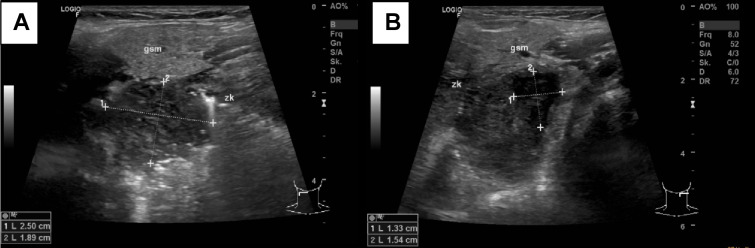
Transverse sonographic images showing (A) an enlarged heterogeneous right-sided tonsil without signs of an abscess and (B) an enlarged heterogeneous left-sided tonsil with a peritonsillar hypoechoic, partly anechoic mass (1.33 × 1.54 cm) displaying a peritonsillar abscess in a 27-year-old female patient; zk: tongue; gsm: submandibular gland.
